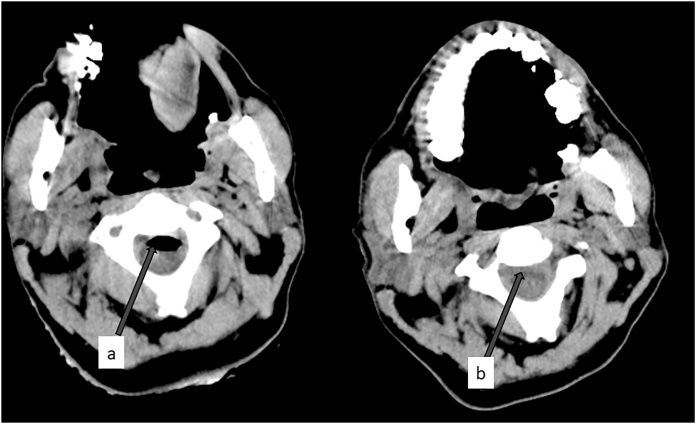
a. Noncontrast axial cervical CT shows pneumorachis in cervical C3 level (red arrow). b. Noncontrast axial cervical CT showed no improvement of pneumorrachis on the C3 level at 72 h (red arrow).
